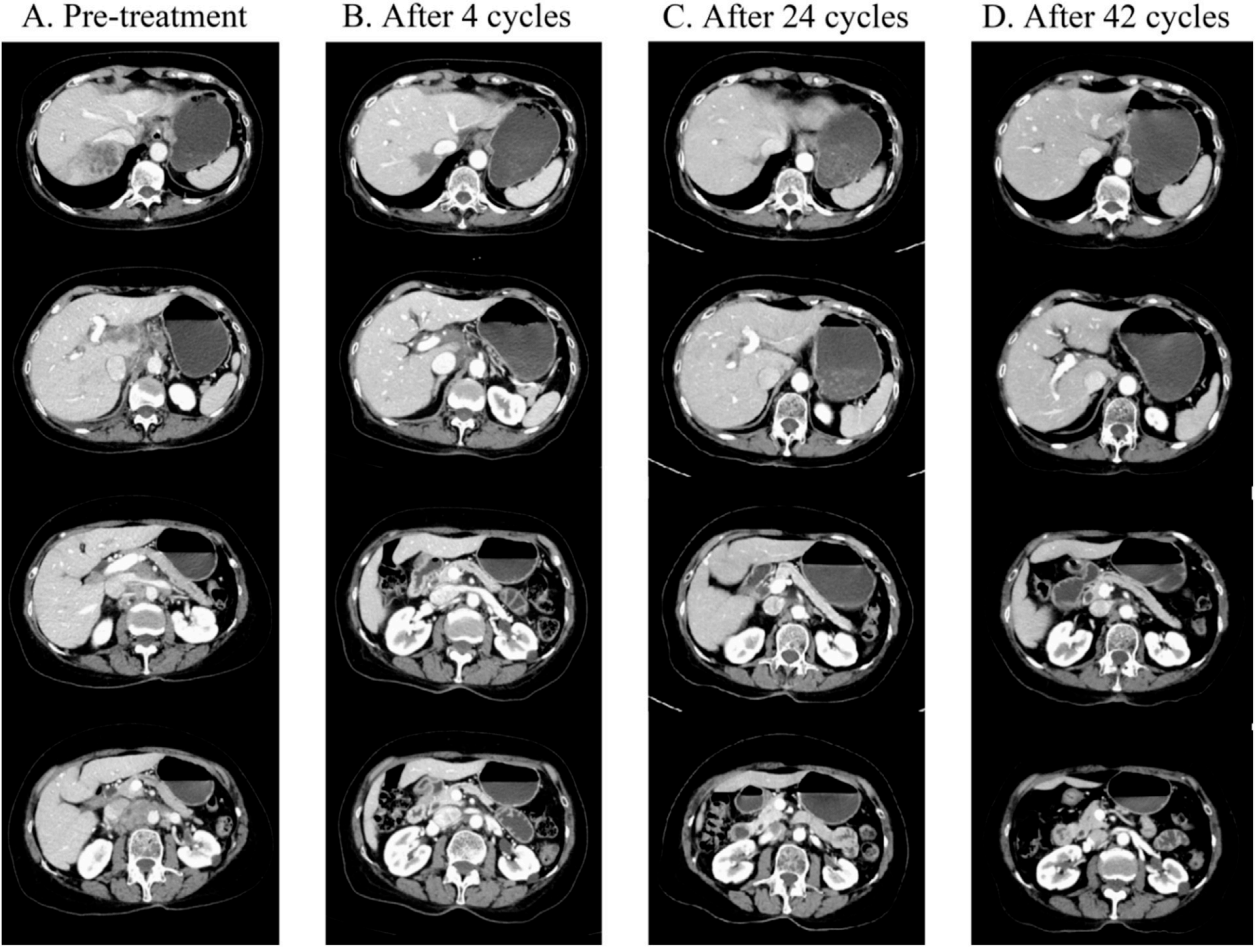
(A) Prior to treatment, CT showed a soft tissue mass located in the right lobe of the liver (size 5.6 cm × 4.7 cm), with increased and enlarged lymph nodes in the perigastric, hepatoportal area, hepatogastric ligament, and para-abdominal aorta, which were partially fused, and most of them were metastatic from lymph nodes (2021-07-12). (B) CT showed that after four cycles of treatment, compared with the 2021-07-12 CT, the primary tumor in the right lobe of the liver was significantly reduced (size 3.1 cm × 3.0 cm), and the caudate lobe lesion was a new metastasis. The hepatic portal area and hepatogastric ligament, cardiophrenic angle area, and para-abdominal aorta metastasis were significantly reduced compared to the previous area. (C) CT on 2022-11-29 showed that after 24 courses of treatment, the primary tumor in the right lobe of the liver almost disappeared, the residual low-density lesions were most likely to be necrotic, and some metastases in the liver almost disappeared. Compared with before, the size of the hepatic portal area and hepatic and gastric ligaments, cardiopulmonary angle area, and para-abdominal aortic lymph nodes continued to shrink. (D) CT on 2024-01-05 shows that after 42 courses of treatment, all lesions have almost completely disappeared compared with 2022-11-29 CT.
